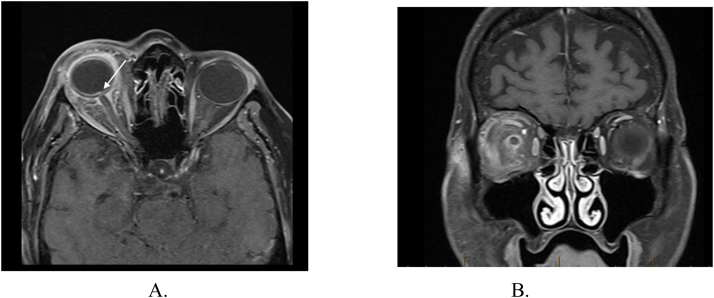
Orbital MRI in the axial (A) and coronal (B) T1- SEFS post-gadolinium administration sequence, showing a right panophthalmitis with associated optic neuritis (papillitis) and orbital cellulitis. Diffuse enhancement of sclera and ciliary body, vitreous heterogeneity with diffuse enhancement pre-septal and post-septal orbital fat was noted, with important conjunctival edema. Enhancement of the optic nerve head (white arrow) of the retrobulbar optic nerve sheath. Retrobulbar optic nerve itself was normal. No signs of an orbital collection, cavernous sinus syndrome or orbital apex syndrome was seen. SEFS: Spin echo fat suppressed.
